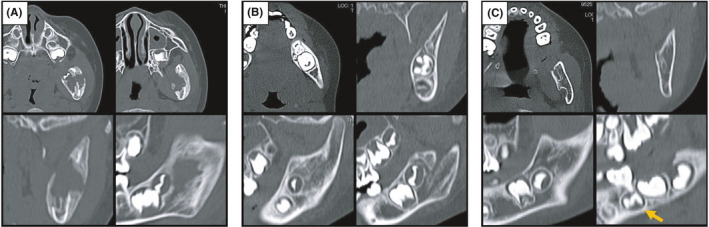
CT images during follow‐up at 2 mo (A), 6 mo (B), and 18 mo (C) after biopsy. Tooth germ formation of the left lower second premolar was found at a site within the original lesion range (yellow arrow)
